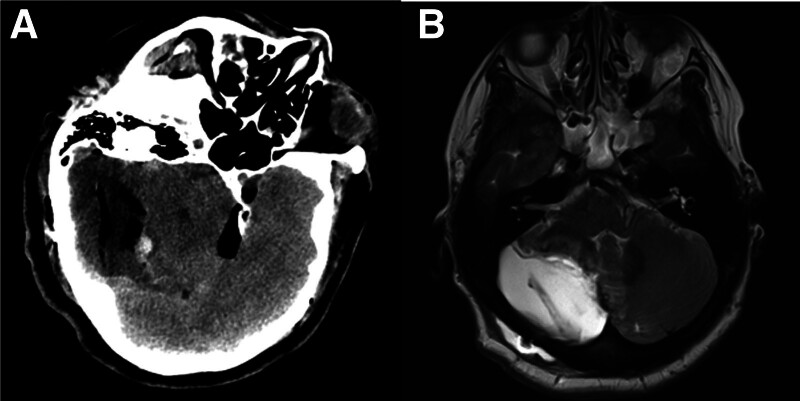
Postoperative CT (A) and MRI (B). CT = computed tomography, MRI = magnetic resonance imaging.
